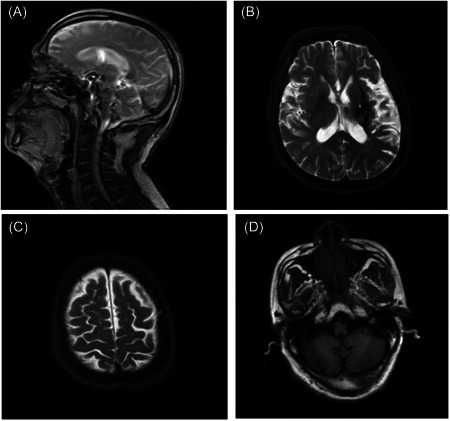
MRI images at admission. MRI showed encephalatrophy, multiple old bleeding, and softening lesions occurred in the left basal ganglia region, coronaradiata and brainstem, bilateral white matter hemorrhage, and demyelinating lesions. MRI, magnetic resonance imaging.
